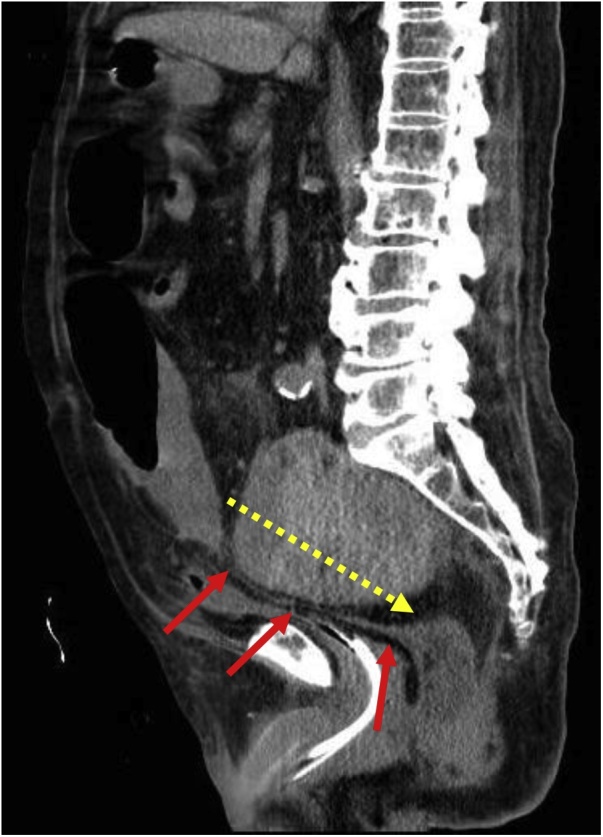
CT findings 10 days after endovascular repair. The rectum is more severely compressed by the aneurysm than before endovascular repair (red arrows). The aneurysm wall was dissected as indicated during open surgery (yellow dotted line).
